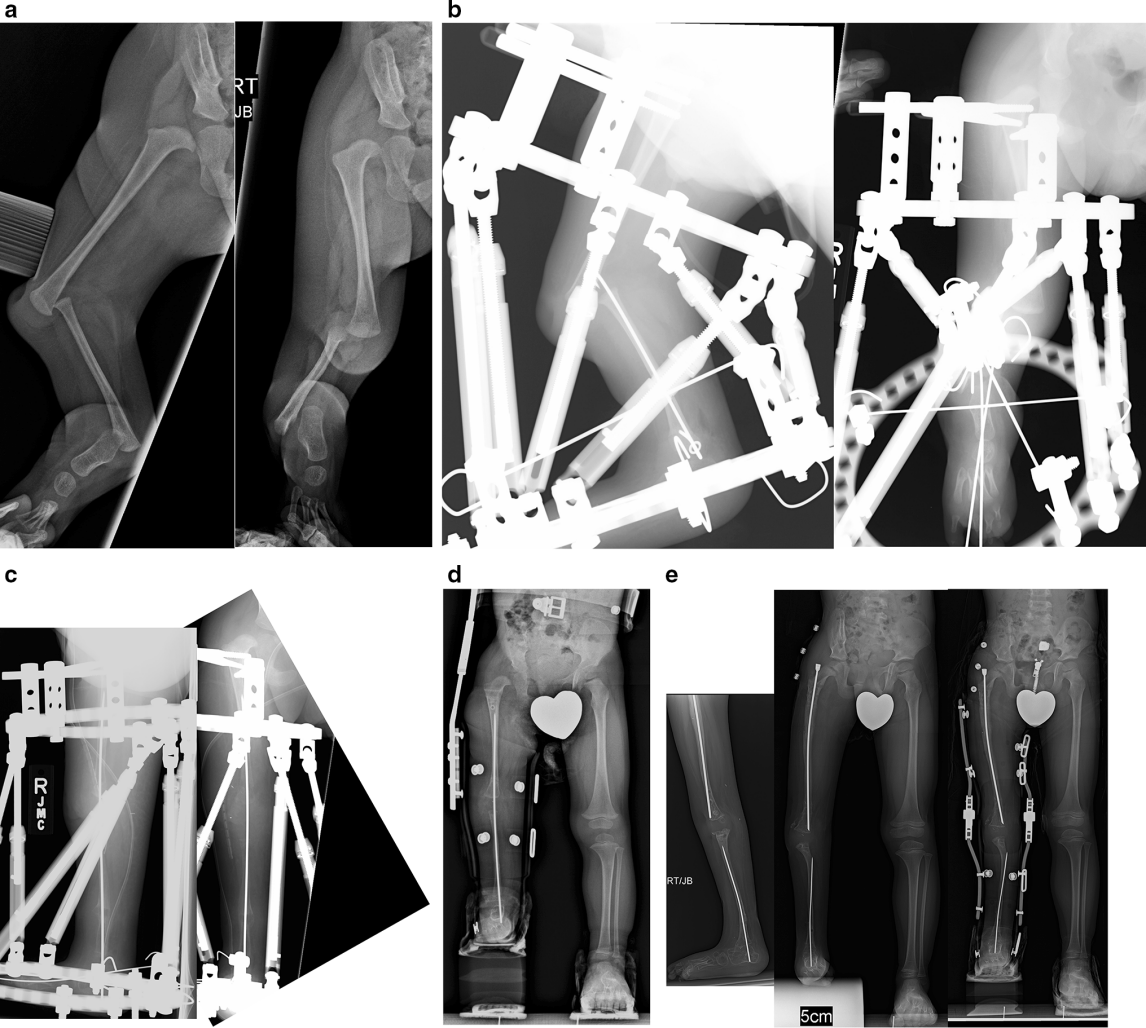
a Preoperative AP (*left*) and lateral (*right*) radiographs of the right leg of a 15-month-old boy born with unilateral tibial hemimelia, Paley type 5C. There is no patella or quadriceps muscle. The knee and ankle have severe contractures. b Lateral (*left*) and AP (*right*) radiographs showing TSF in place with one ring on femur and one on the foot. There is a temporary epiphysiodesis wire in the fibula hooked at both ends. There is a transverse wire through the fibula to transport it distally. c Intraoperative (hemovac seen) lateral (*left*) and AP (*right*) radiographs after complete correction of the knee and ankle and after the second staged surgery to fuse the ankle and to temporarily arthrodese the knee using an axial wire. Tendon transfers were also done to replace the absent quadriceps muscle. d AP standing radiograph following removal of external fixator 3 months later. The axial wire was left in place to protect the knee for 6 more months. There is excellent alignment with significant leg length difference. e Standing lateral (*left*) and AP (*middle*, *right*) radiographs at age 5 yers, after lengthening of the femur and tibia and after a pelvic osteotomy was done to stabilize the hip. Separate intramedullary wires remain in place to protect the bones from fracture and to guide hypertrophy. A KAFO is used for several years—until the knee becomes stable—to allow knee flexion and extension while protecting varus and valgus bending on the hypertrophying knee joint for several years until the knee becomes stable
